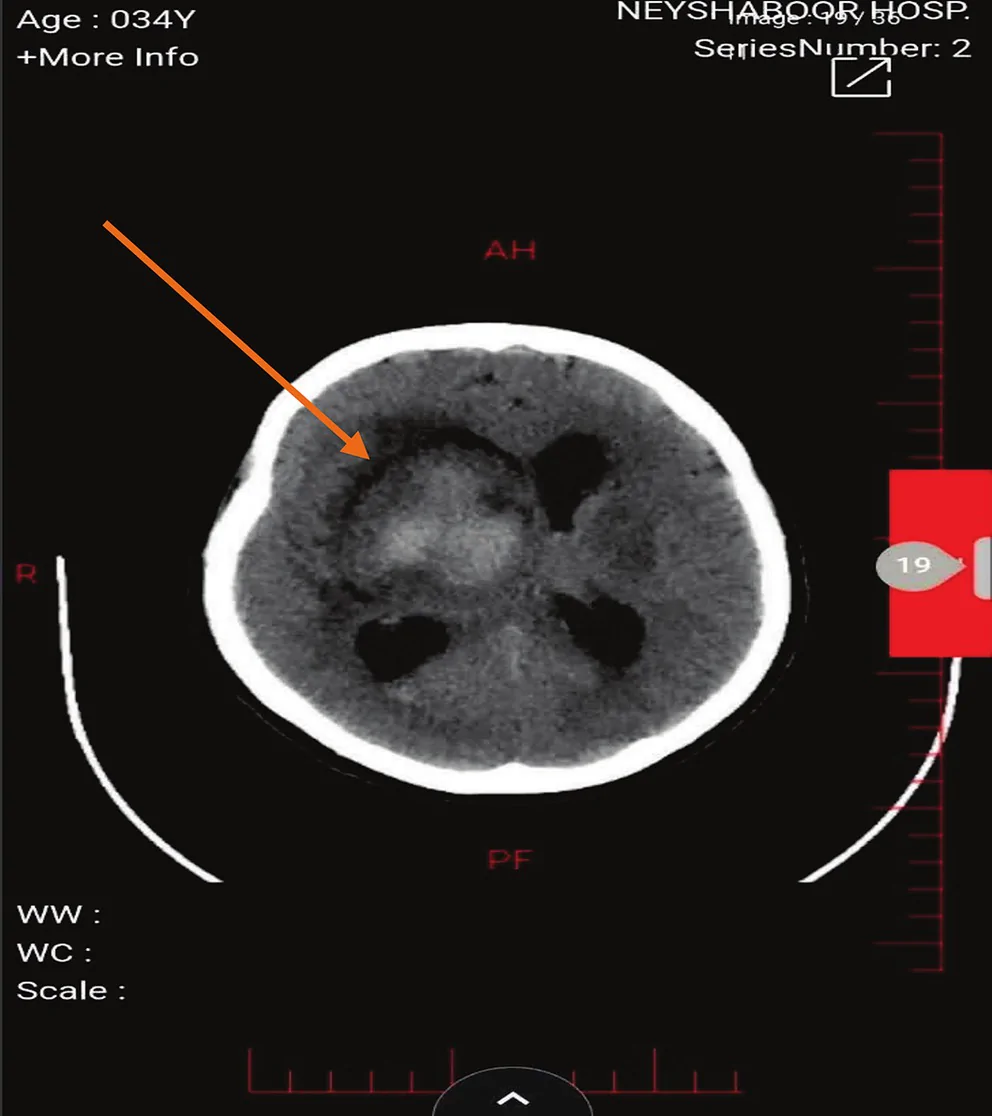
Non‐contrast CT scan of the head showed extensive ICH.
